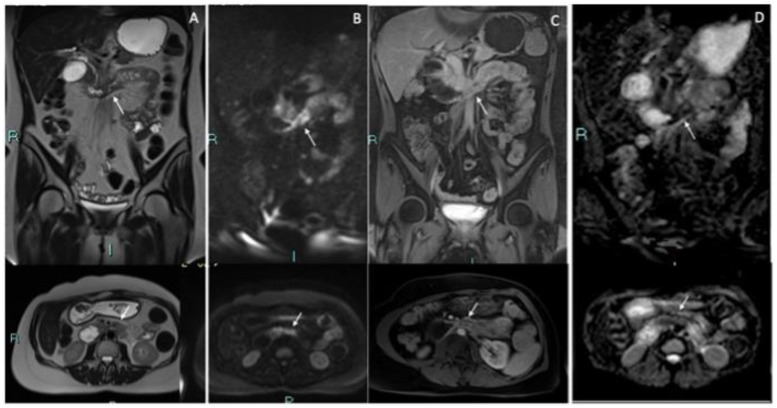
Pre-operative MRI (Magnetic Resonance Imaging) examination was per Figure 2. w-haste images and axial T2w-haste images showed severe stenosis of the third duodenal portion. (B,C) DWI (Diffusion Weighted Imaging) (A) and ADC (Apparent Diffusion Coefficient) (B) sequences coronal and axial images showed an evident restriction of diffusivity due to the marked increase in cellularity in the neoplastic tissue (D). Coronal and axial T1 and post-contrast (gadolinium) images showed marked contrast impregnation of the wall. There was no significant biliary dilatation.
